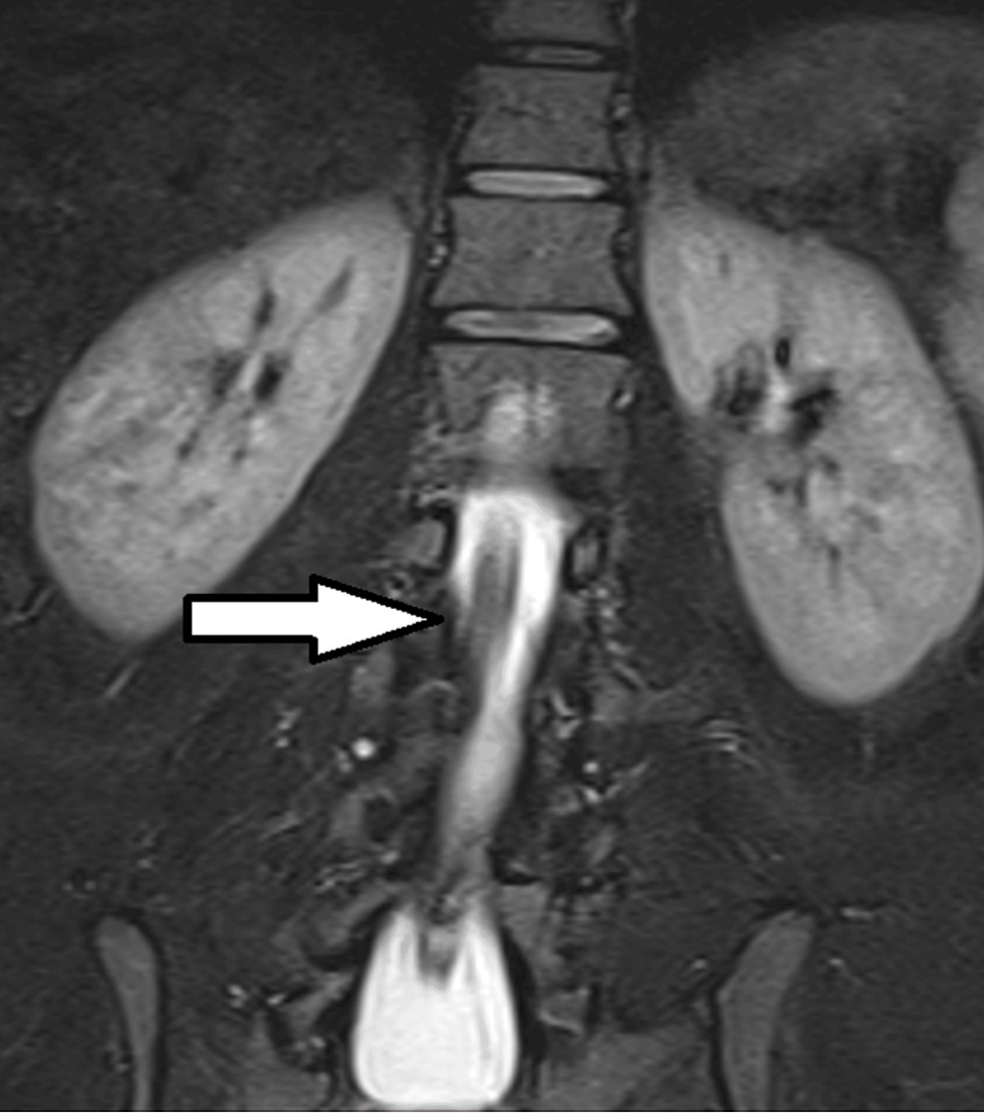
MRI of diastematomyelia and mild scoliosis in L4-L5 sections of the spinal cord (coronal plane) Note the bony spur dividing the hemicords (arrow).
